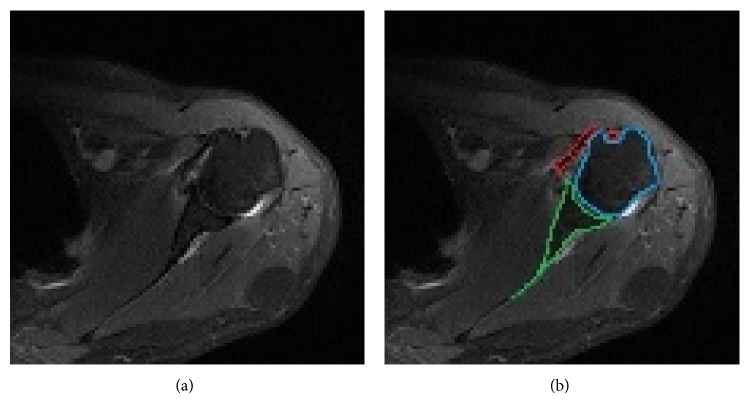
(a) Axial PD weighted MRI of shoulder; (b) biceps and subscapularis tendons are demonstrated in red area. The boundary of scapula and humeral head is colored in green and blue, respectively.
